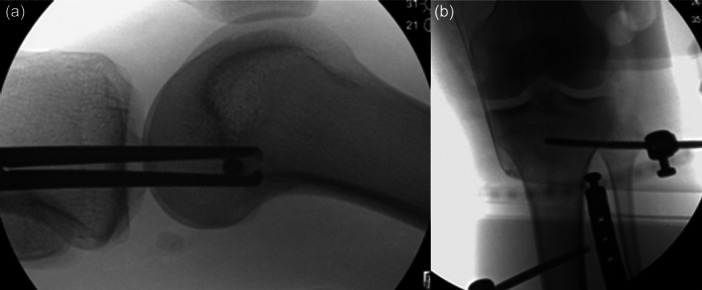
(a) Lateral fluoroscopic image demonstrating the placement of the central axis reference pin off of which the hinged external fixator will be based. (B) AP radiograph demonstrating the hinged external fixator in place after the central axis reference pin has been removed. AP, anterior–posterior.
